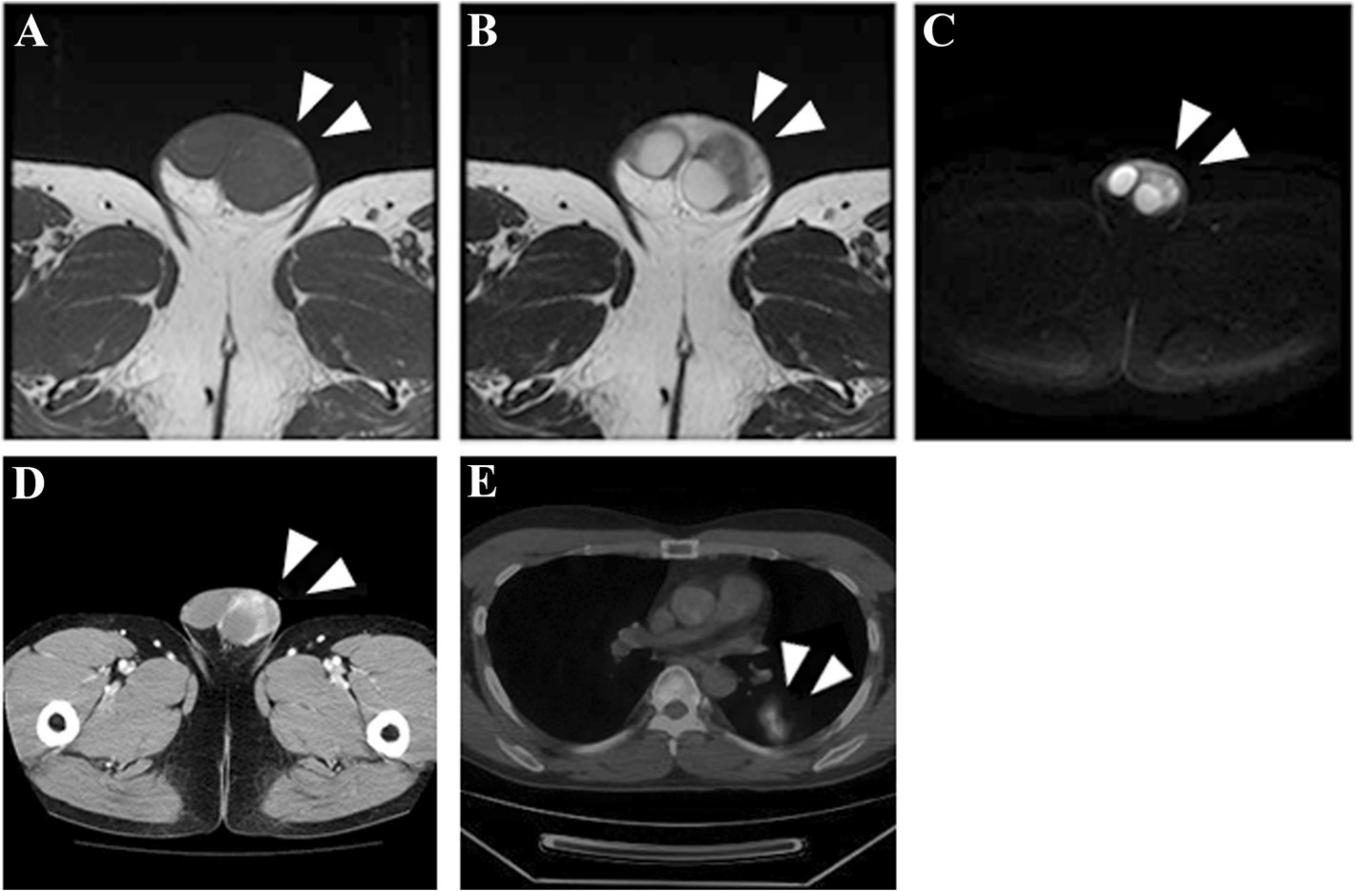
Pre-operative diagnostic imaging. Magnetic resonance imaging of the left scrotum shows a low-intensity mass in the paratesticular region on T1 (A) -and T2 (B) -weighted imaging, and areas of high signals on diffusion-weighted imaging (white arrows; C). *The paratesticular lesion is irregularly enhanced on CT imaging*
